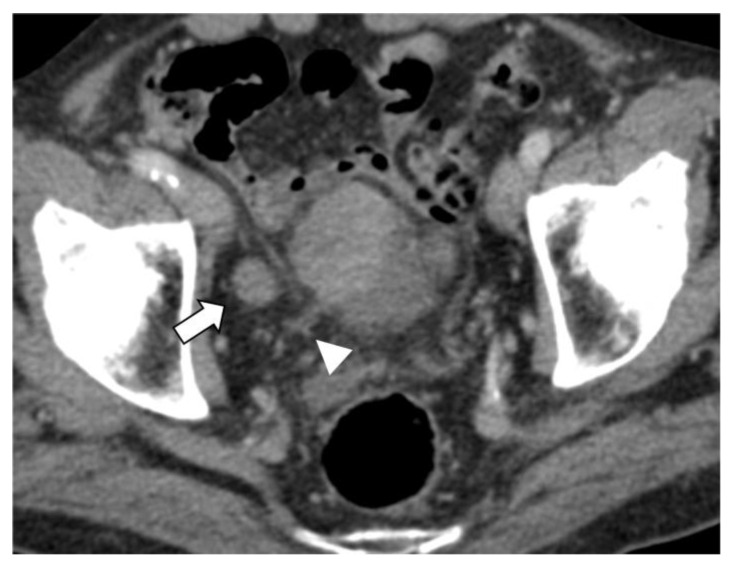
Bladder cancer, stage pT3 pN3. Presence of a metastatic LN with a short axis of 16 mm (arrowhead) and LVD of 5.6 mm (arrowhead) on the axial CT venous phase.
